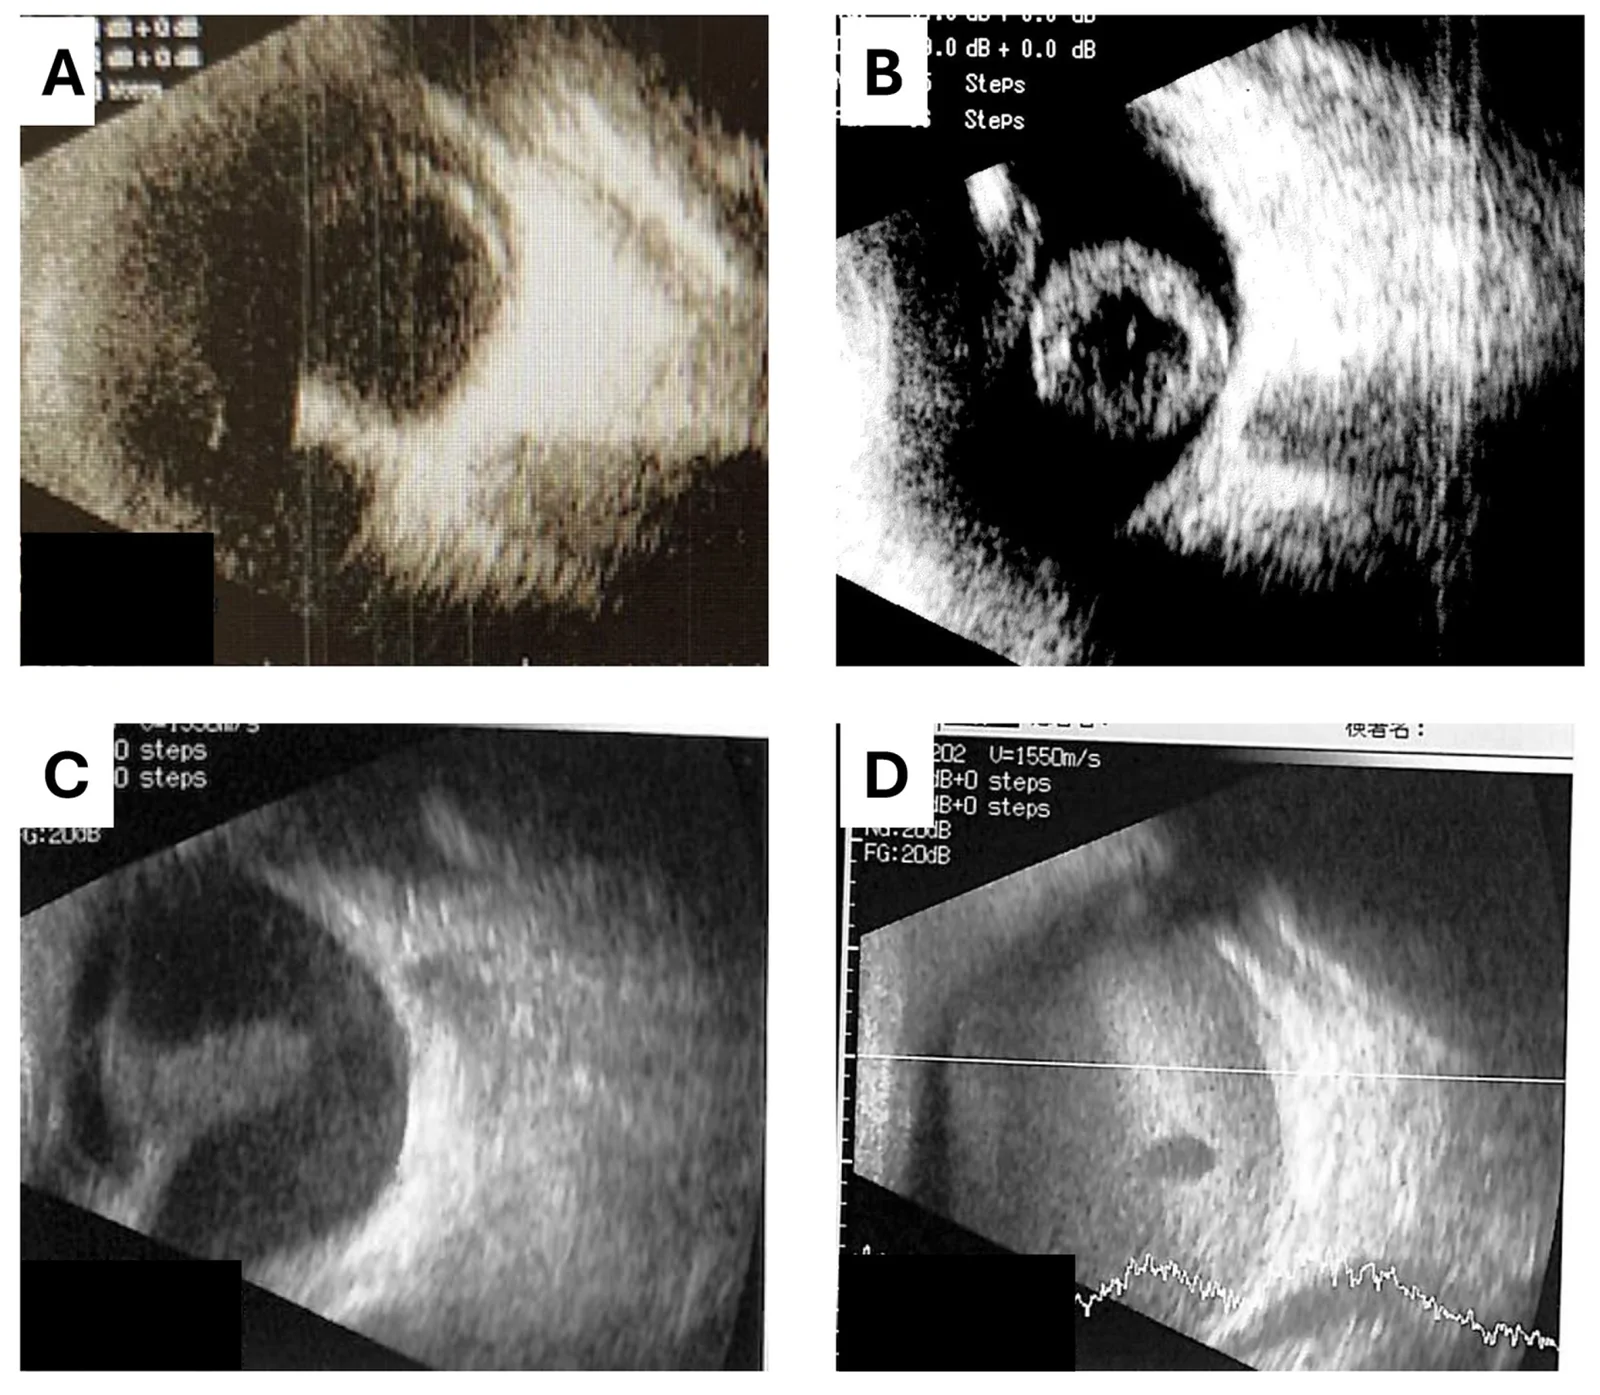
Representative B-scan ultrasonography findings in Norrie disease. (A–D) B-scan ultrasonography images from the right eye of Patient 11 (A), the right eye of Patient 5 (B), and the left (C) and right (D) eyes of Patient 3. (A) Partial retinal detachment associated with a PFV-like stalk. (B,C) Open- and closed-funnel retinal detachments, respectively. (D) Diffuse high echogenicity caused by advanced vitreoretinal degeneration and subretinal hemorrhage, precluding accurate assessment of the retinal configuration.
